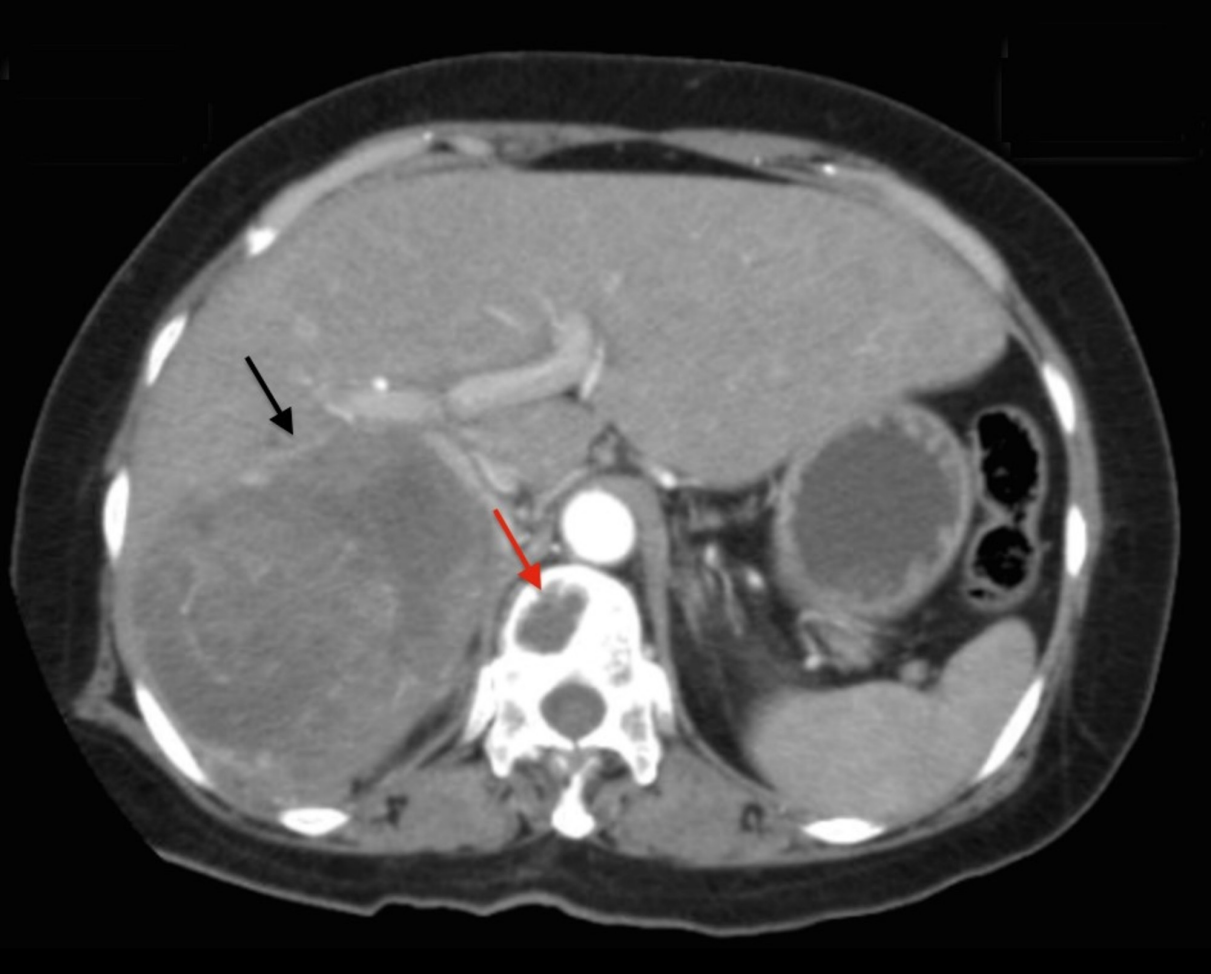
Axial section at the level of T12 from the CT scan of the patient showing the adrenal mass (black arrow) and a lytic lesion in the vertebra (red arrow) CT: computed tomography
